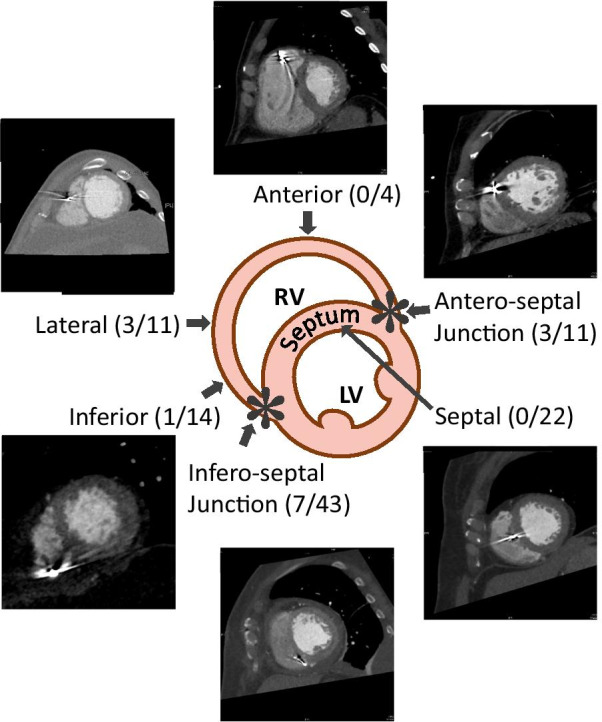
Anatomical distribution of subclinical perforation by screw-in right ventricular lead in the short axis view of cardiac CT
